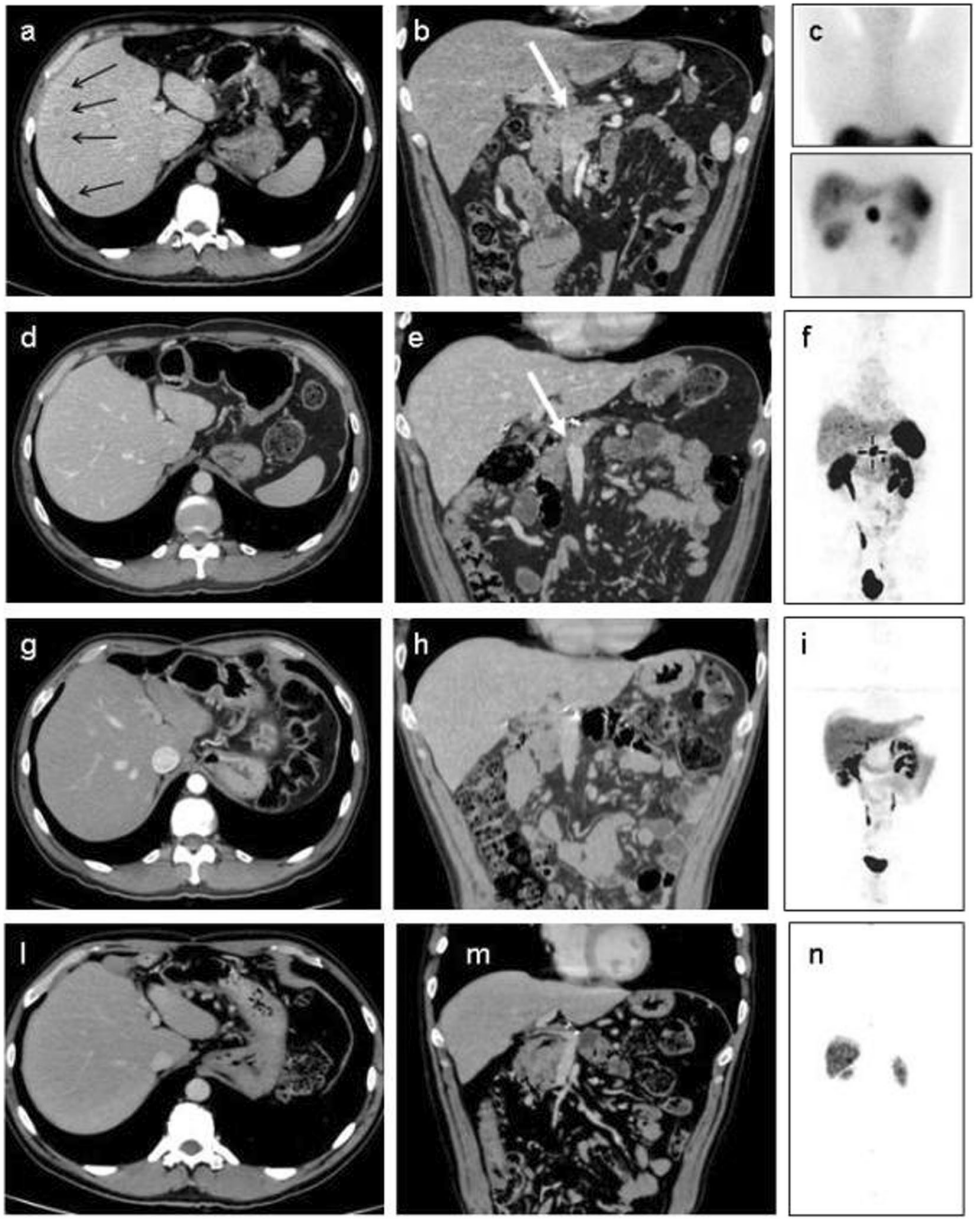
Clinical case imaging. Imaging at the time of diagnosis. Axial portal venous phase CT (a) shows multiple metastases in the right liver lobe (*arrows*). Multiplanar coronal reconstruction (b) shows a 4 cm mass (*white arrow*) in the pancreatic body. Anterior planar Octreoscan scan (c) confirms the pathological intense tracer uptake in the pancreatic tumor and liver metastases. The patient provided written informed consent for disclosing clinical and imaging data. Follow-up imaging 5 months after PRRT. Axial portal venous phase CT scan (d) shows the absence of liver metastases and the reduction (>50%) in the pancreatic mass (*white arrow*) (e). Anterior planar ^68^Gallium-DOTANOC PET-CT scan (f) confirms the absence of the pathological uptake in the liver and a radiotracer uptake reduction in the pancreatic tumor. g–i Imaging 3 months after surgery. Axial CT scan (g) shows post-operative findings of distal pancreatectomy and splenectomy with a persistent absence of liver metastases. On coronal CT image (h), the pancreas head is free of local recurrence. Anterior planar ^68^Gallium-DOTANOC PET-CT scan (i) confirms the absence of the pathological uptake both in the liver and in the residual pancreas. Imaging ~10 years after surgery. Axial-coronal CT scans (l, m) and anterior planar ^68^Gallium-DOTANOC PET-CT scan (n) overlap with the previous morpho-functional imaging and show the absence of recurrence both in the liver and in the residual pancreas
